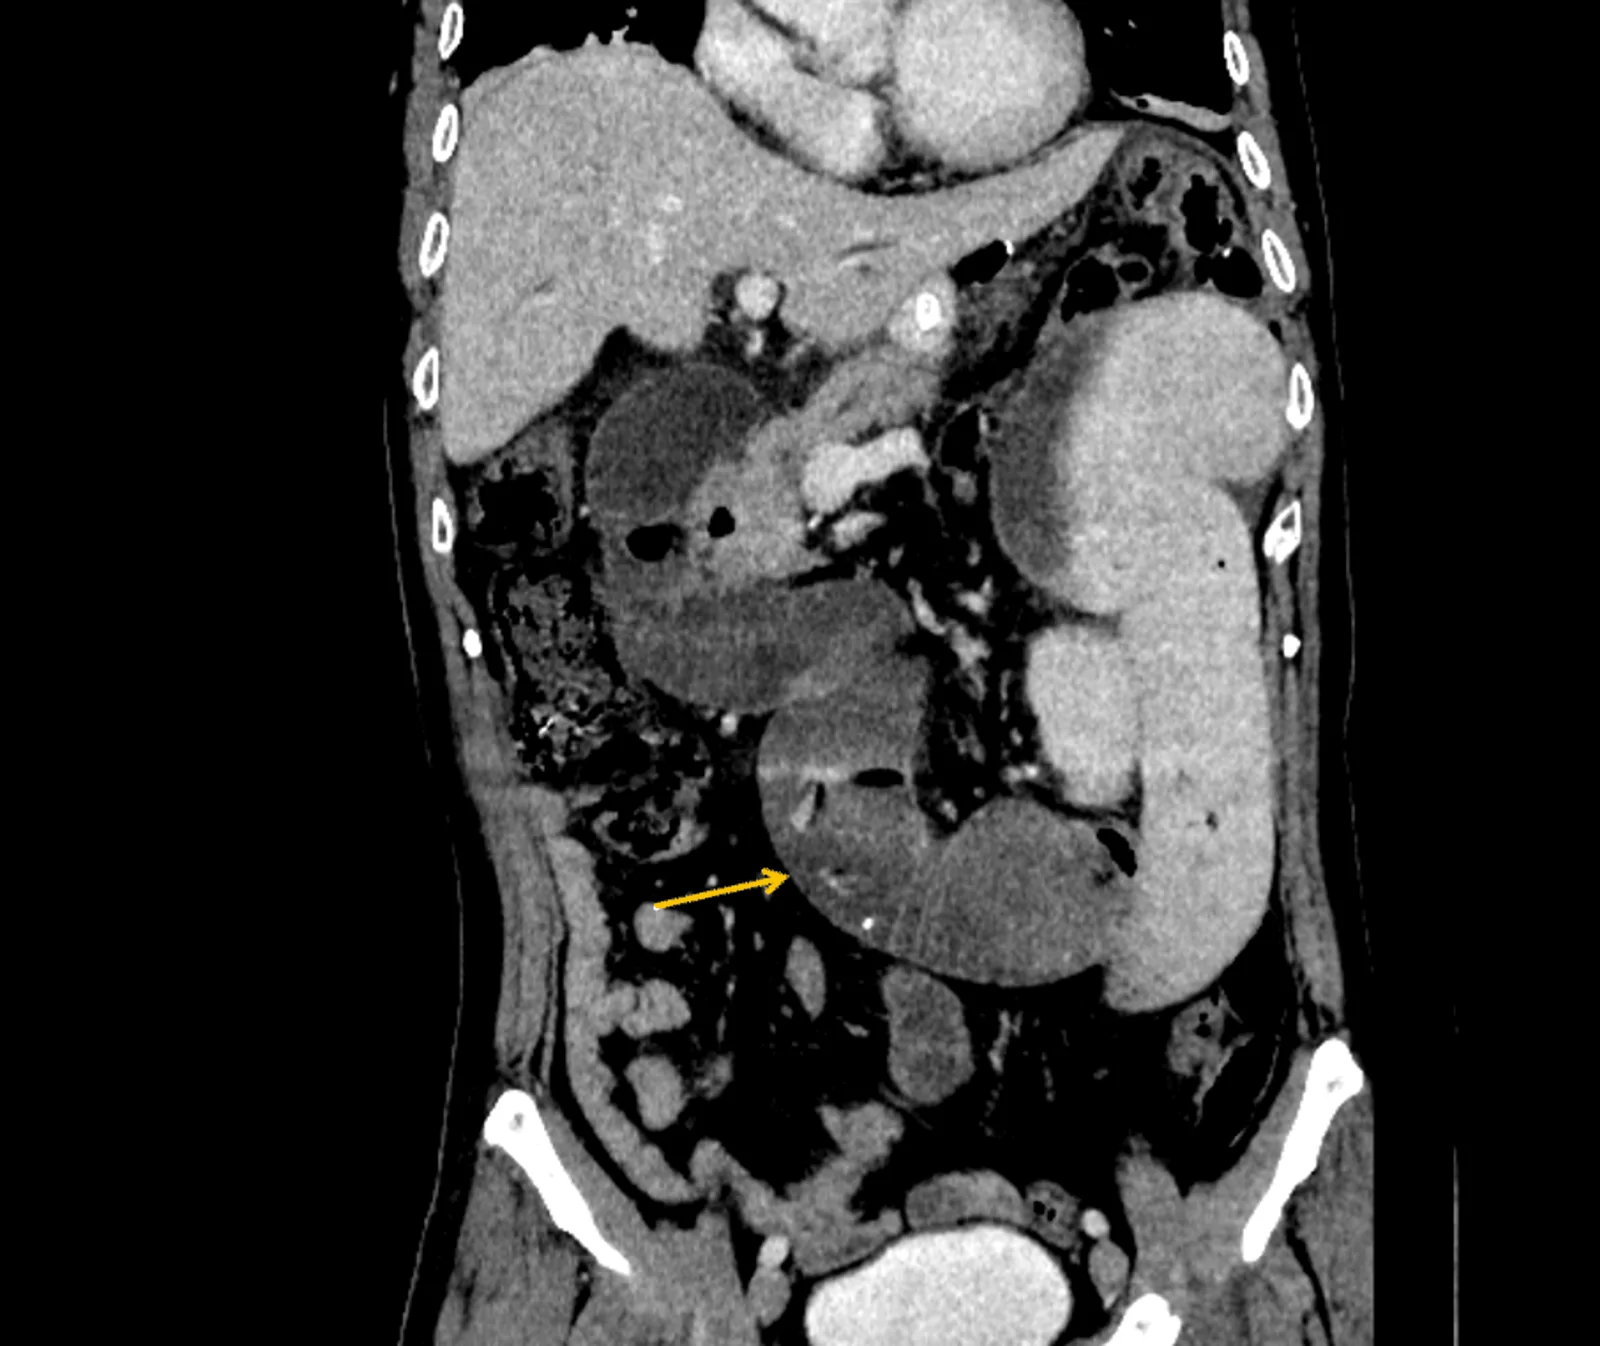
Coronal contrast-enhanced CT performed approximately 72 hours after symptom onset demonstrating dilatation of the duodenum and afferent jejunal limb (yellow arrow). Distal ileal loops are collapsed, while orally administered contrast reaches only the proximal ileum.
